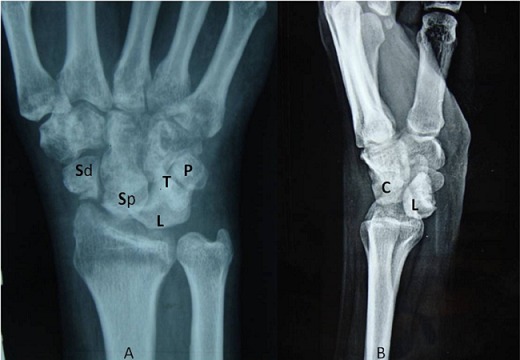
Radiographs showing (a) dislocated proximal pole of scaphoid (Sp), lunate(L), triquetrum(T) and pisiform(P) in PA view, (b) showing volar subluxation of the lunate in lateral view
